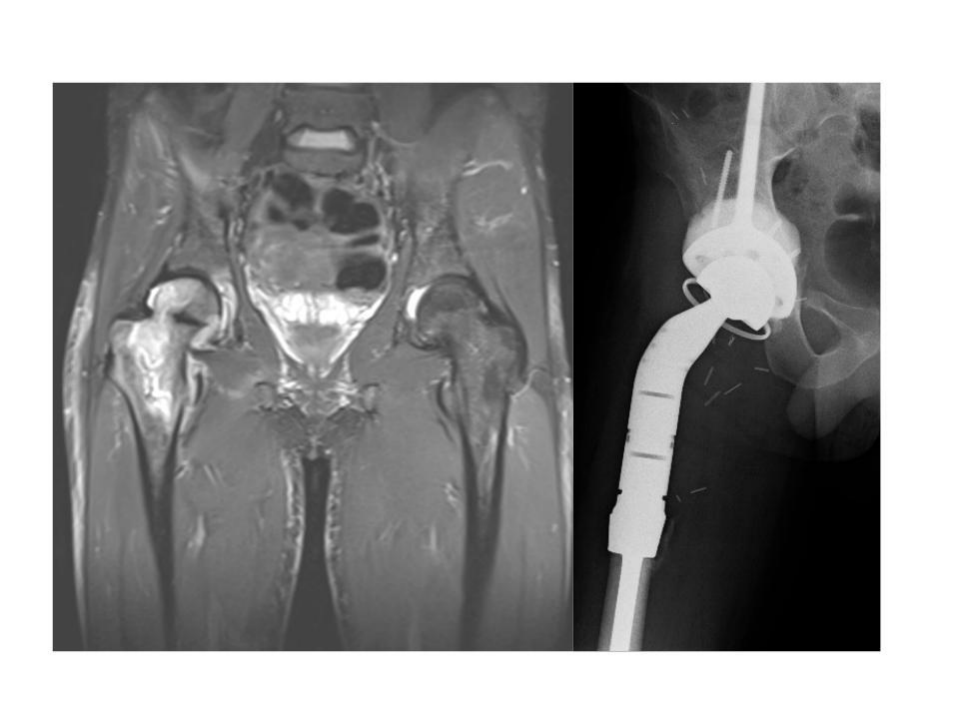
Preoperative MRI and postoperative x-ray of the pleomorphic sarcoma reconstructed with a coned pelvis.
